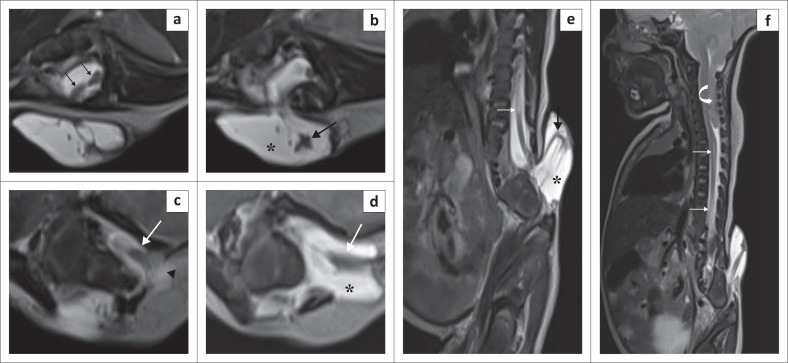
Type II diastematomyelia with a right hemimyelomeningocoele, left hemilipomyelomeningocoele and Arnold Chiari II malformation. Axial T2-weighted image (T2WI) (a) shows the splitting of spinal cord (diastematomyelia) (thin black arrows). Axial and sagittal T2WI (b & e) show herniation of the right hemicord (solid black arrows) along with an enlarged subarachnoid space (*) through the right posterior bony defect with no overlying skin or subcutaneous tissue (right hemimyelomeningocoele). Axial T2WI (c) shows herniation of the left hemicord (solid white arrow) through the left posterior bony defect with tethering to a subcutaneous lipomatous mass (black arrowhead). Axial T2WI (d) shows protrusion of the neural tissue and an enlarged CSF space (*) through the bony defect (left hemilipomyelomeningocoele). Sagittal T2WI (f) shows herniation of the cerebellum (white curved arrow) into the spinal canal through the foramen magnum and syrinx formation (thin white arrow).
